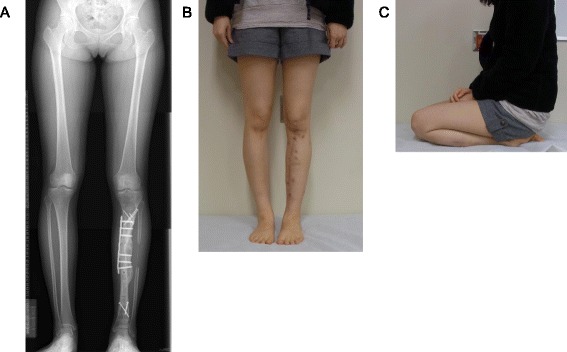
Follow-up radiography and photography. a Radiograph taken 2 years after the fourth surgery shows the complete union of the osteotomy site and normal alignment of the left leg. b Clinical photo taken from an anteroposterior view demonstrates the normal appearance of the leg. c Clinical photo taken from a lateral view shows the normal functioning of the left knee joint
